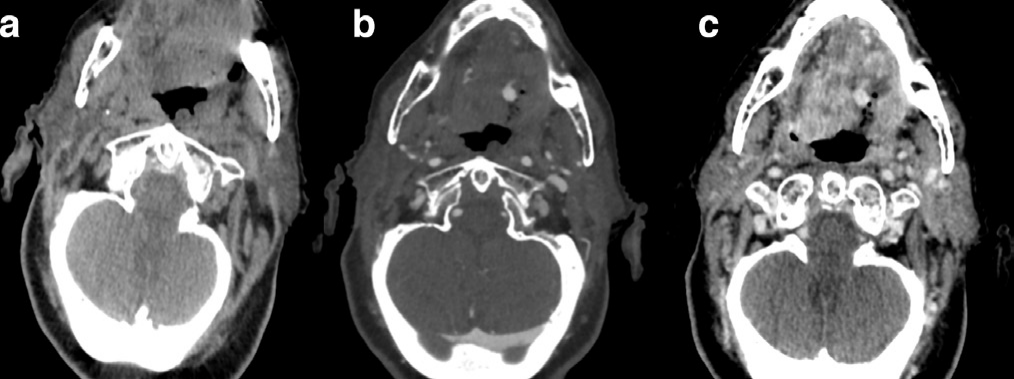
Transverse images of unenhanced (A), angiographic (B) and venous (C) CT phases show a large ulcerative mass at the base of the tongue with a pseudoaneurysm within, and no obvious contrast media extravasation.
